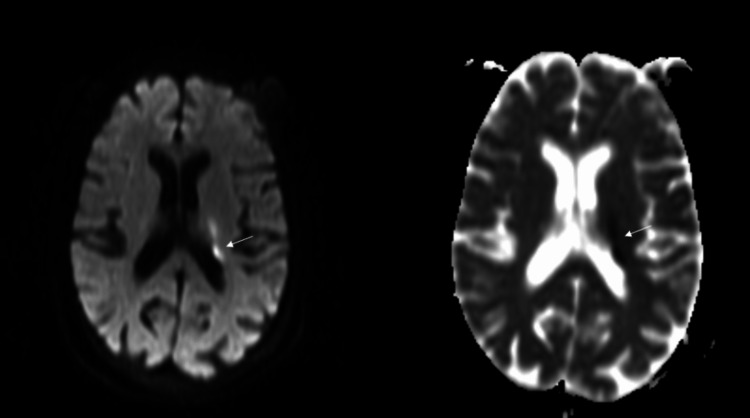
DWI showing diffusion restriction in the left corona radiata (white arrow on left) with corresponding low signal on ADC (white arrow on right). ADC, apparent diffusion coefficient; DWI, diffusion-weighted imaging
